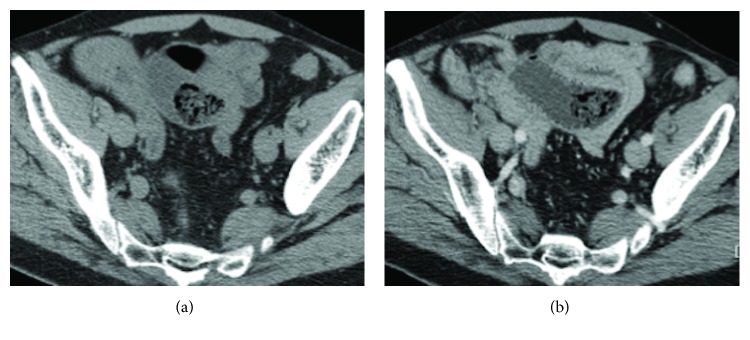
Axial CT scan of a 60-year-old man previously operated of cholecystectomy and splenectomy. Unenhanced phase (a) shows dilatation of the jejunal loops, but a neoplastic origin could not be ruled out. Post contrast image in the portal venous phase (b) shows absence of pathologic enhancement of the bowel wall, and the final diagnosis of small bowel obstruction due to adhesions was made.
